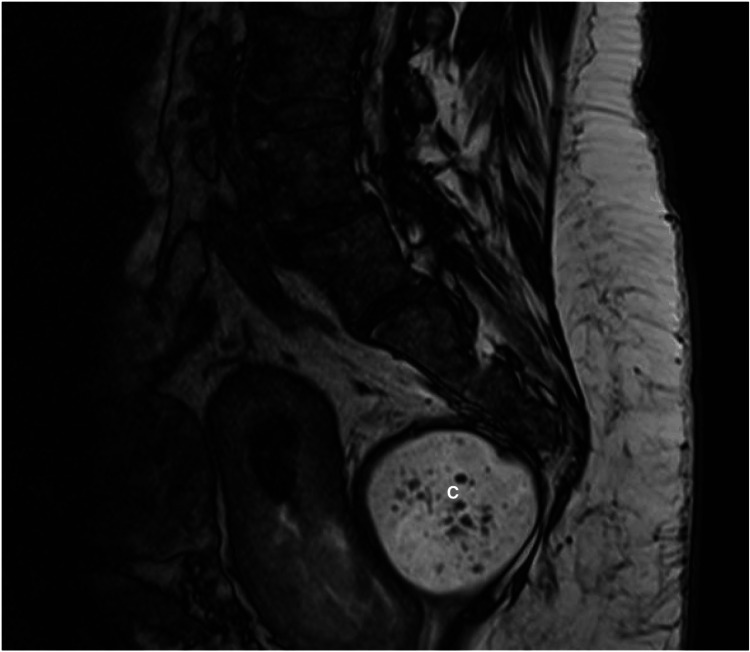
Preoperative magnetic resonance imaging of recurrent teratoma measuring 61 mm × 48 mm × 57 mm (C) (T2, Sagittal Slice 2019).
